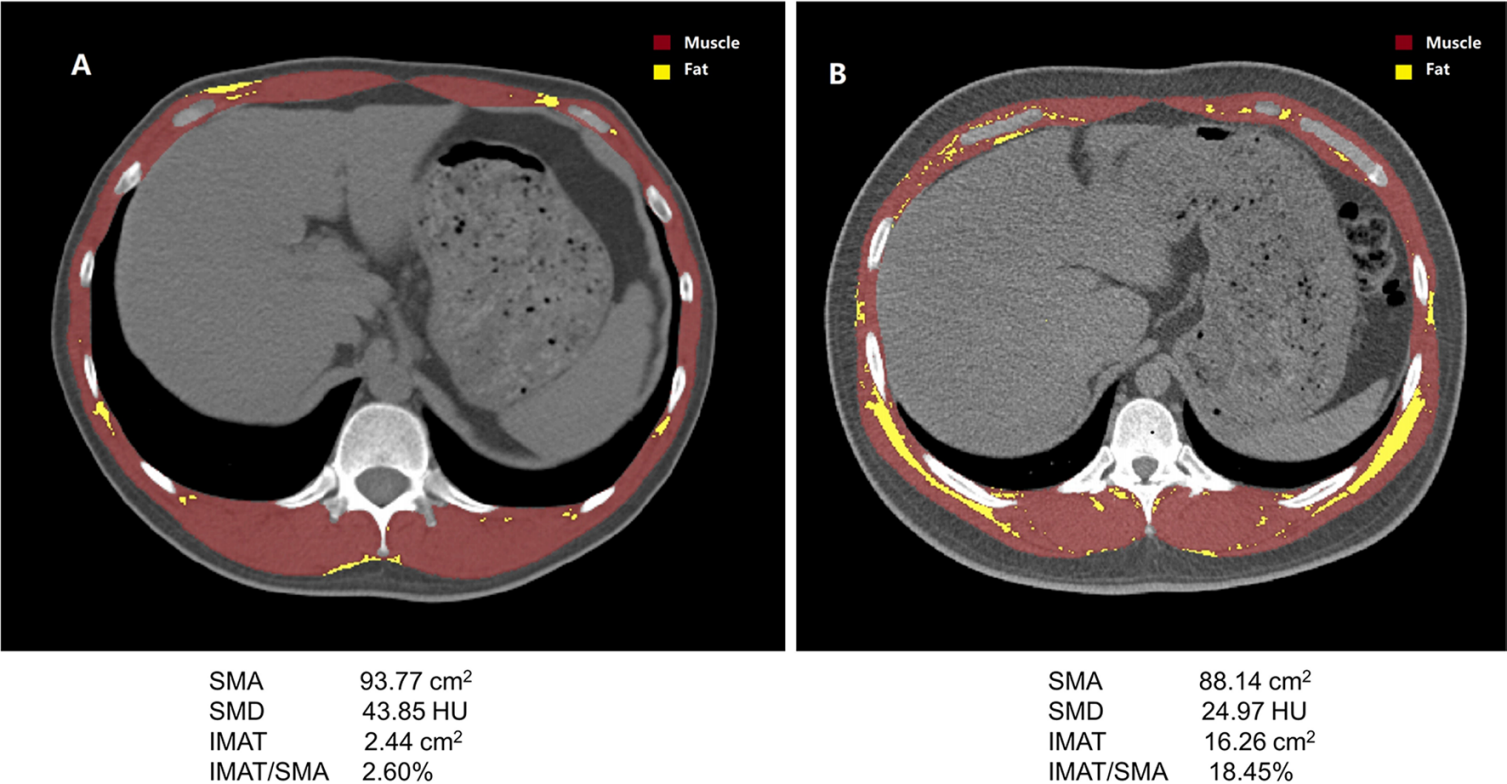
Cross-sectional CT images at the T12 level used for the quantification of muscle mass and fat mass in a 39-year-old man (A) and a 62-year-old woman (B)
